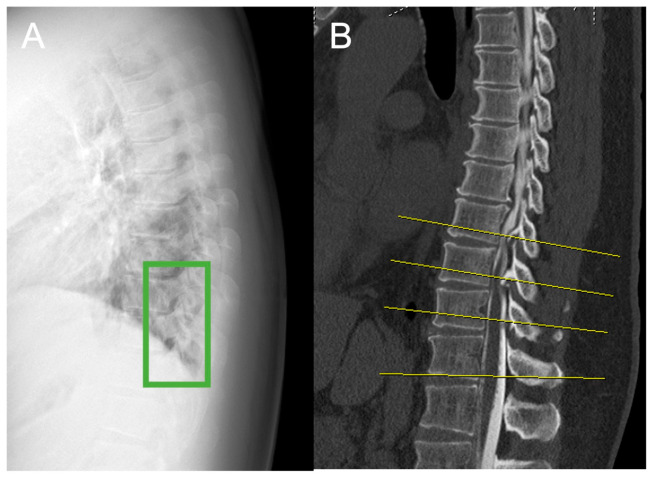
Preparation of images for training the object detection model. Images were annotated with a label by manually inputting a minimal bounding box (a green box) containing the OPLL and OLF on the thoracic lateral radiographs after the exact location of the OPLL was confirmed by CT to generate an image for the object detection training by one orthopedic spine surgeon (15 years): (A) dataset image of a plain lateral thoracic spine radiograph; (B) sagittal plane CT image to identify OPLL. CT: computed tomography; OPLL: ossification of the posterior longitudinal ligament, OLF: ossification of the ligamentum flavum.
